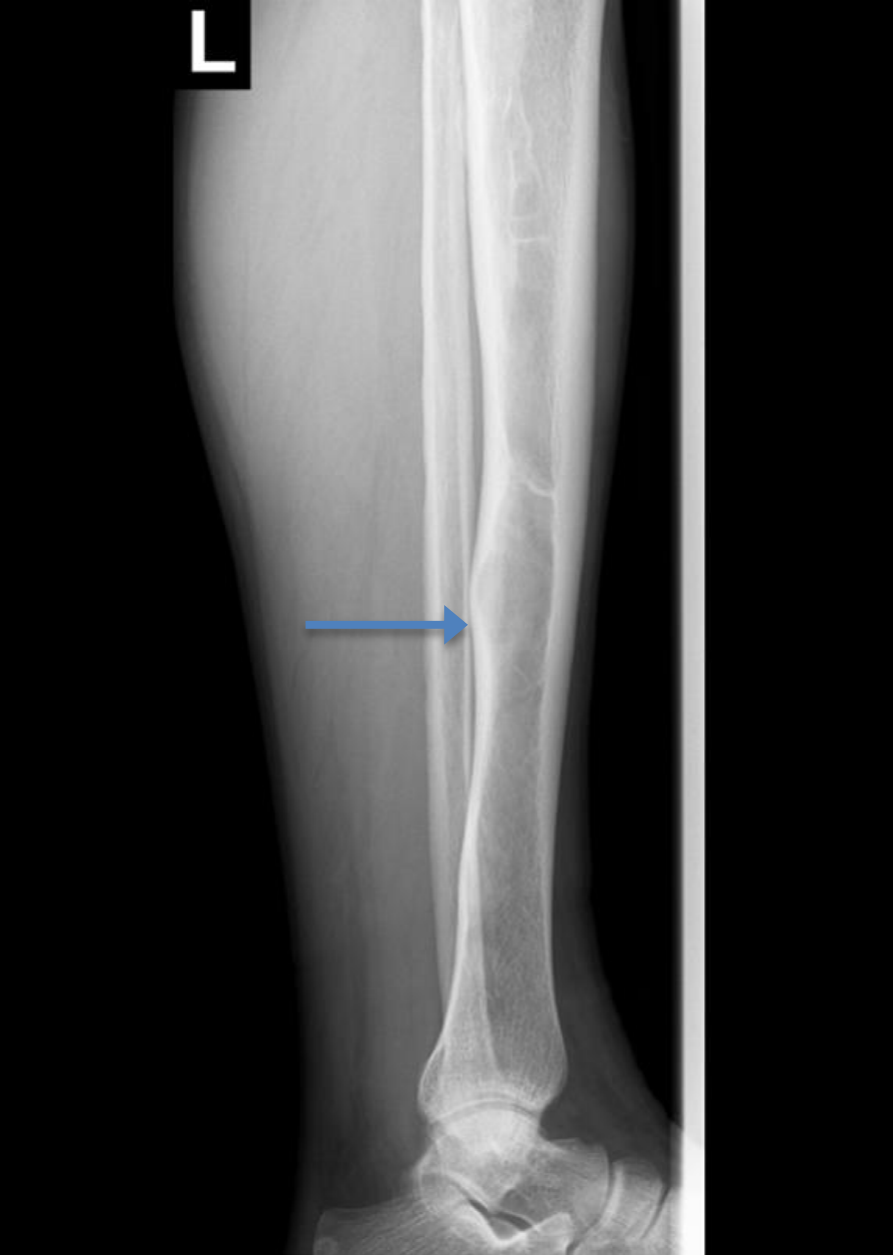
Tibial radiograph showing bone dysplasia in the middle and distal parts of the diaphysis
